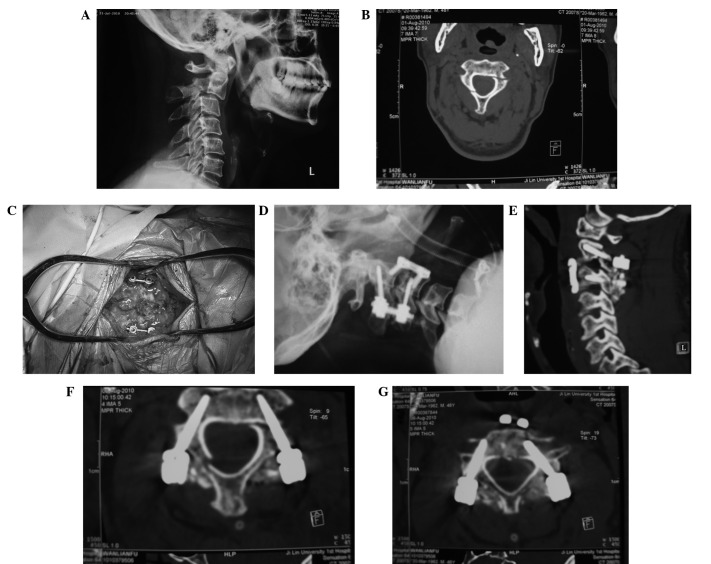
Hangman’s fracture type IIA. (A) X-ray film showing a type IIA fracture; (B) CT scan showing the fracture form; (C) intraoperative screw placement; (D) postoperative lateral X-ray film; (E) postoperative vertical CT; (F) transverse C2; and (G) transverse C3. CT, computed tomography.
